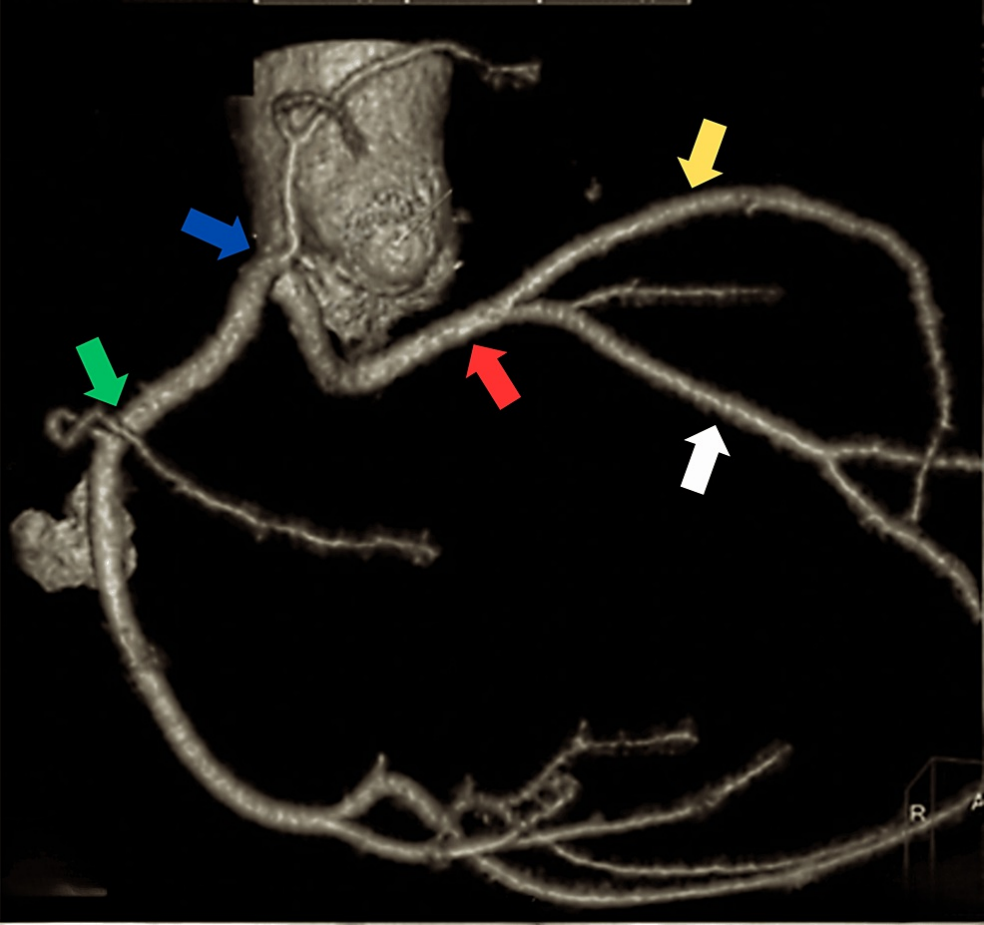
A computed tomography angiogram showing anomalous left (red arrow) and right (green arrow) coronary arteries arising from a single sinus (blue arrow), LAD artery (yellow arrow), and LCX artery (white arrow) LAD: left anterior descending, LCX: left circumflex
